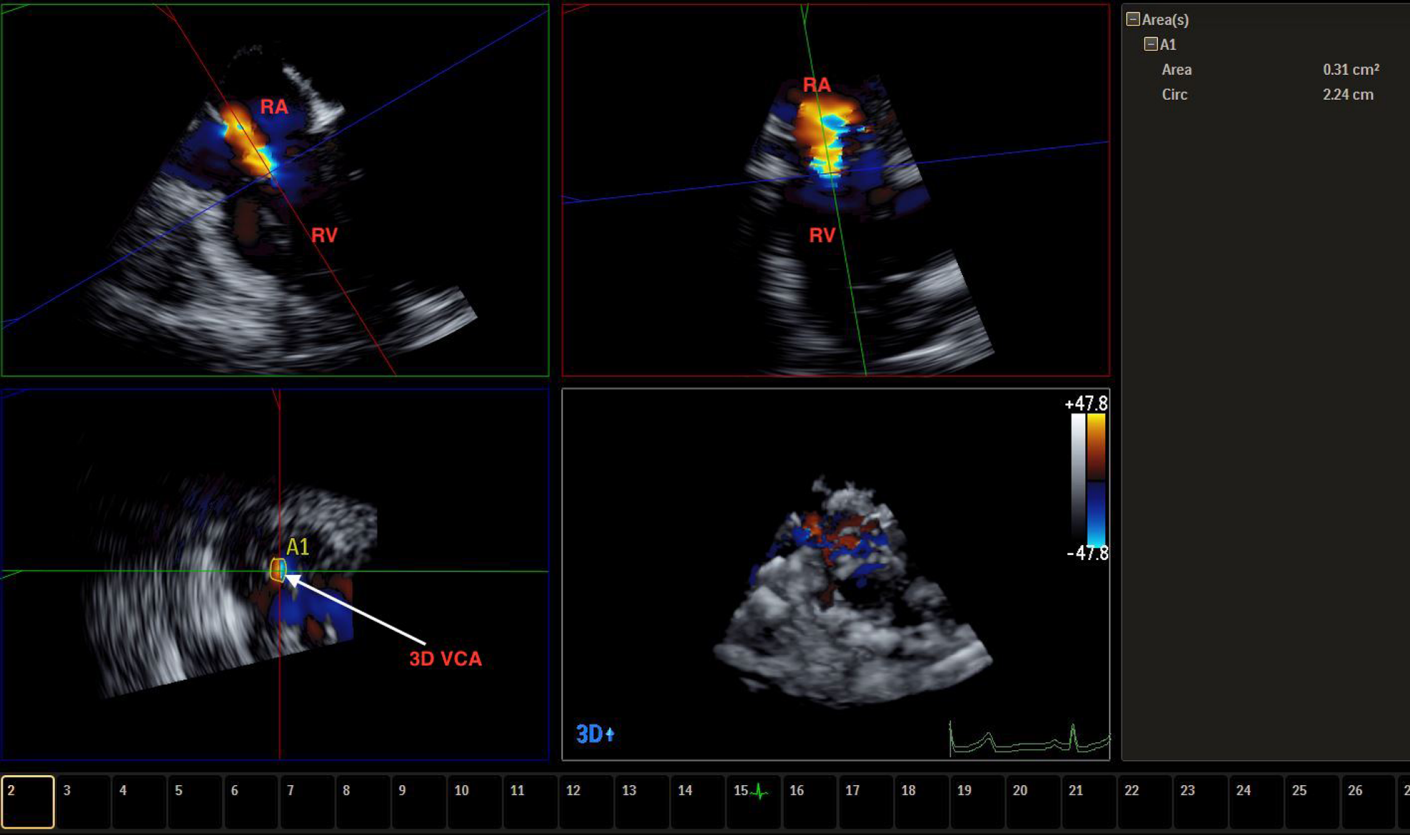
Live/real time transesophageal echocardiographic assessment of TR. The color Doppler data set obtained from a four chamber view was cropped to depict the VC which measured 0.41 cm^2^ in area (A). Abbreviations as in previous figures.
